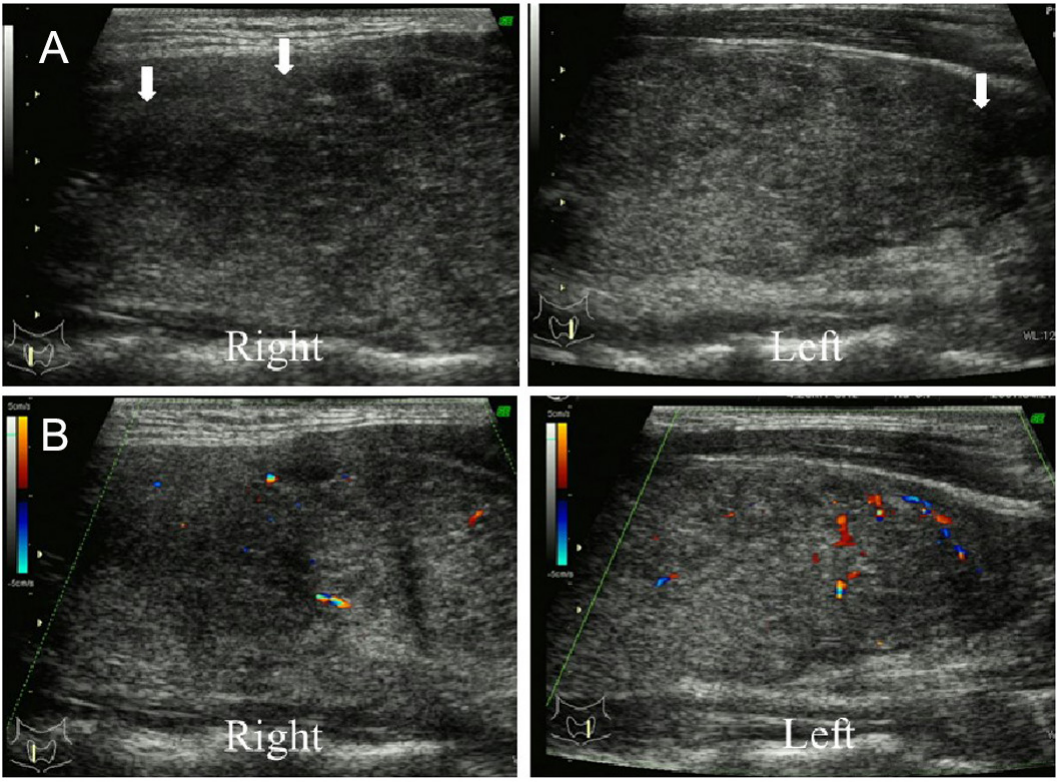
A) Sagittal ultrasonography of the thyroid gland during the initial visit to our hospital in case 1. B) Color-flow Doppler ultrasonography of the thyroid gland in case 1.
